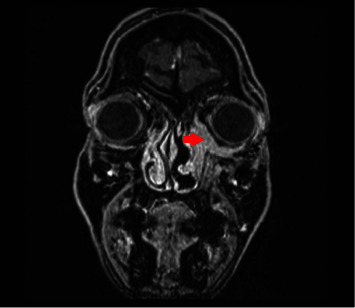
MRI coronal view revealing a lobulated mass with an anteroposterior diameter of 42 mm and a thickness of 13 mm located at the left lateral nasal wall and infiltrating the medial and lower border of the orbit without evident muscle infiltration (red arrow).
